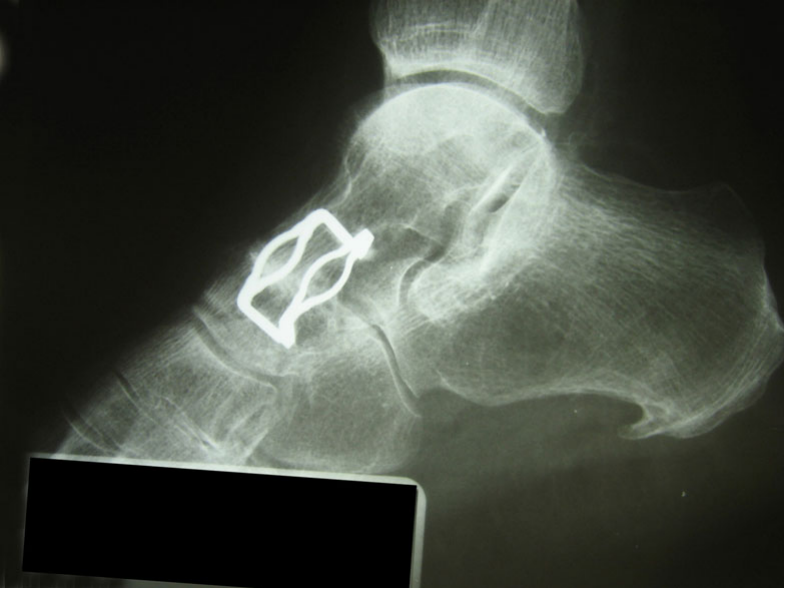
The fixation of arthrodesis by two memory staples on X-ray. Radiograph shows the healed arthrodesis three years after the procedure.
